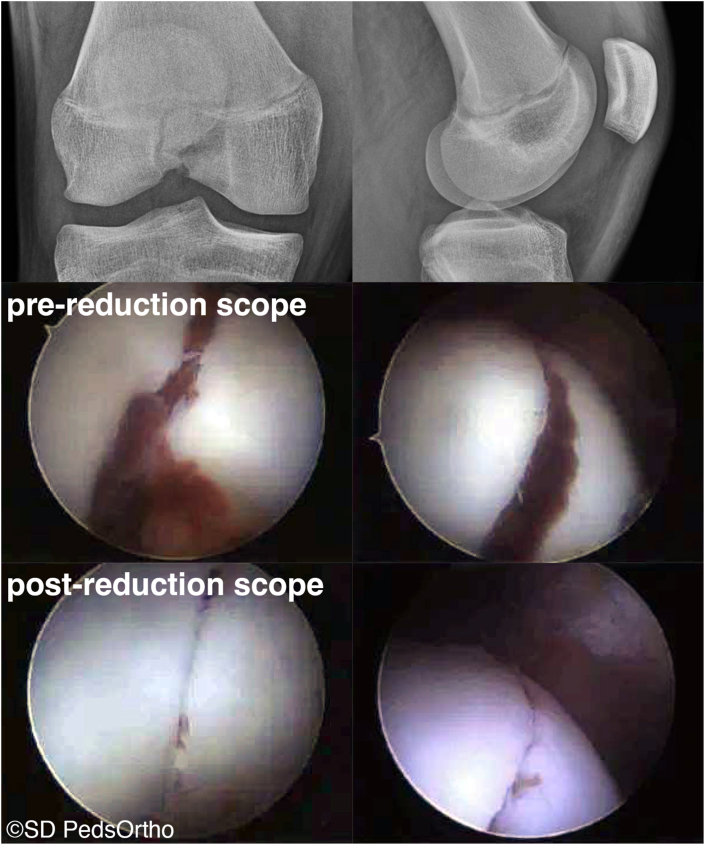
Injury radiographs from 16-year-old male. Arthroscopic images showing the fracture before reduction (top row, left near the notch and right across the condyle) and images following the presented reduction maneuver (bottom row representing the juxtaposed top row image).
